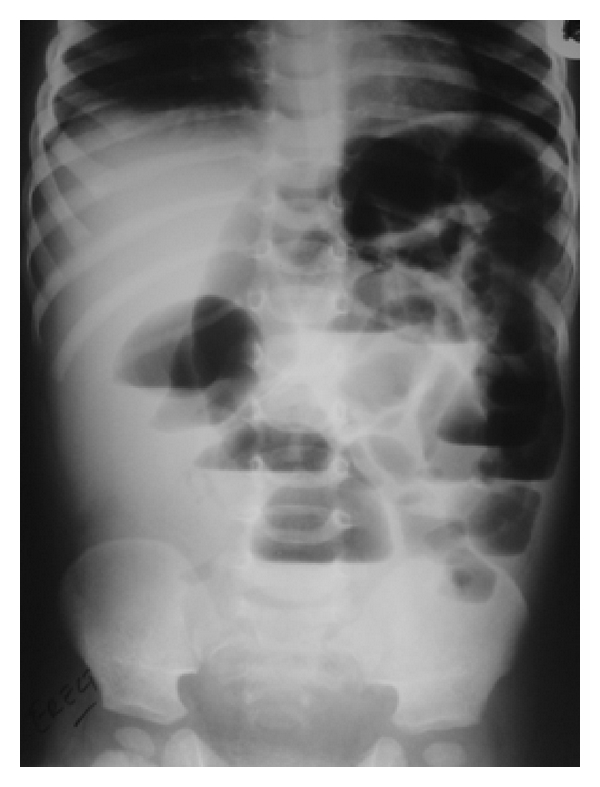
A plain abdominal X-ray shows small bowel intestinal obstruction.
